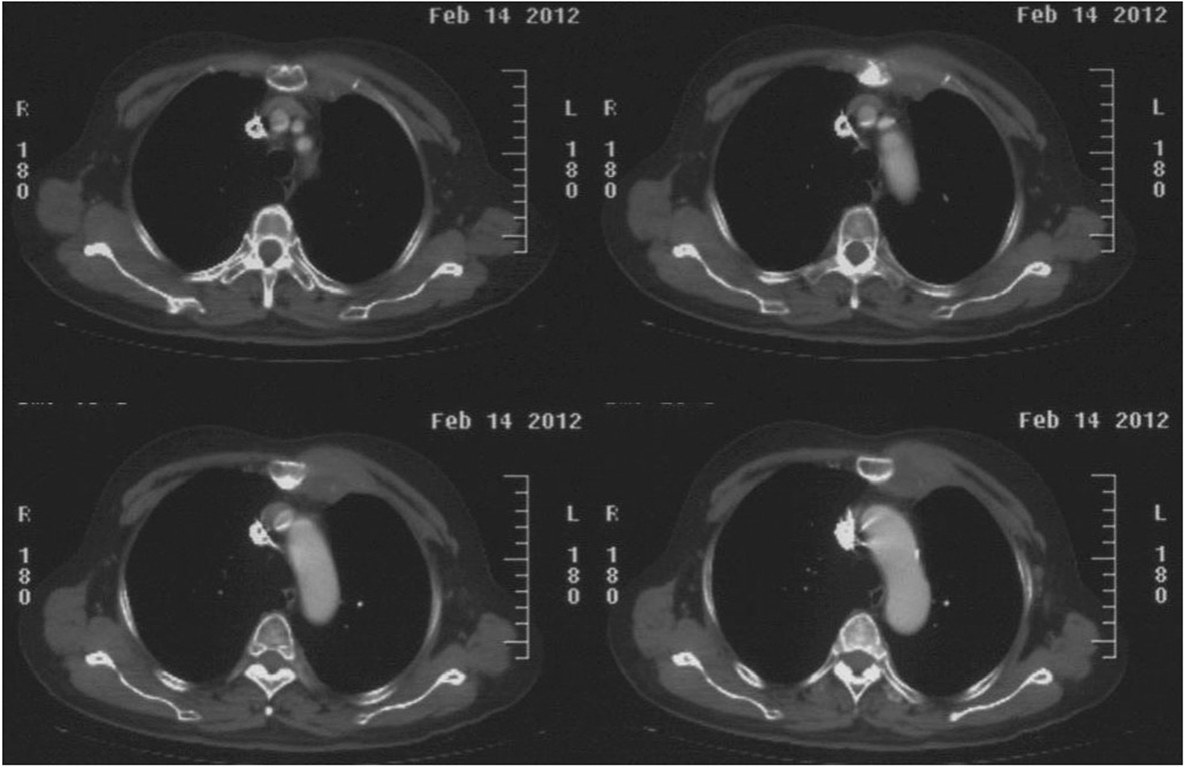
Computed tomography scan showing a solid, round mass in the left anterior chest wall.
